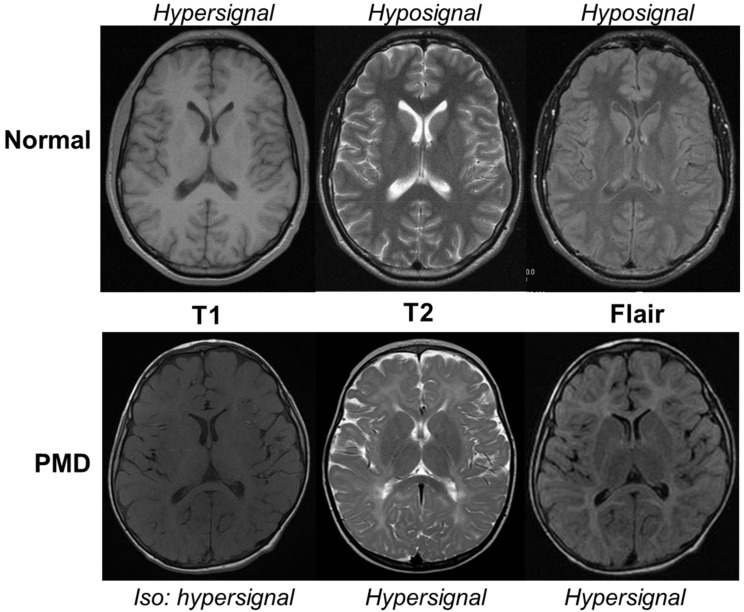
MRI of a 5-year-old Pelizaeus–Merzbacher Disease (PMD) patient in comparison with an age-matched normal boy (Normal). In physiological conditions, the myelinated white matter has a hypersignal T1, hyposignal T2, and flair when compared to gray matter. In PMD, the hypomyelinated white matter has a normal hypersignal T1 appearance contrasting with an abnormal hypersignal T2 and flair. The corpus callosum is partially myelinated with a hyposignal T2 but not with flair.
